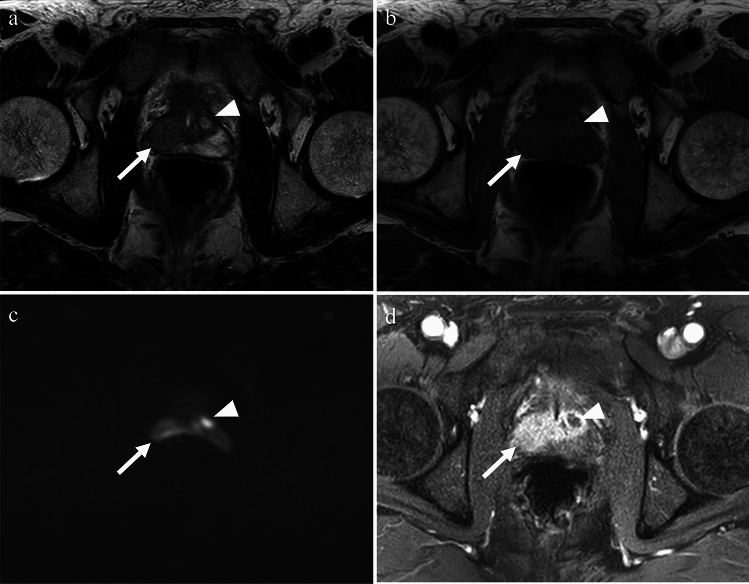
Man in his 60 s with BCG-related infection presenting with dysuria. a On T2-weighted image, a low signal area is observed on the right side of the prostate (arrow), and on the left side, a low signal nodule with high signal intensities in the central portion is noted (arrowhead). b On T1-weighted image, both show slightly higher signal intensities compared to the muscle (arrow and arrowhead). c On diffusion-weighted images, both exhibit strong high signal intensities (arrow and arrowhead). d After contrast administration, there is a diffuse enhancement on the right side, indicative of prostatitis(arrow), while the nodular lesion on the left side shows a contrast defect in the central portion, indicating an abscess (arrowhead)
